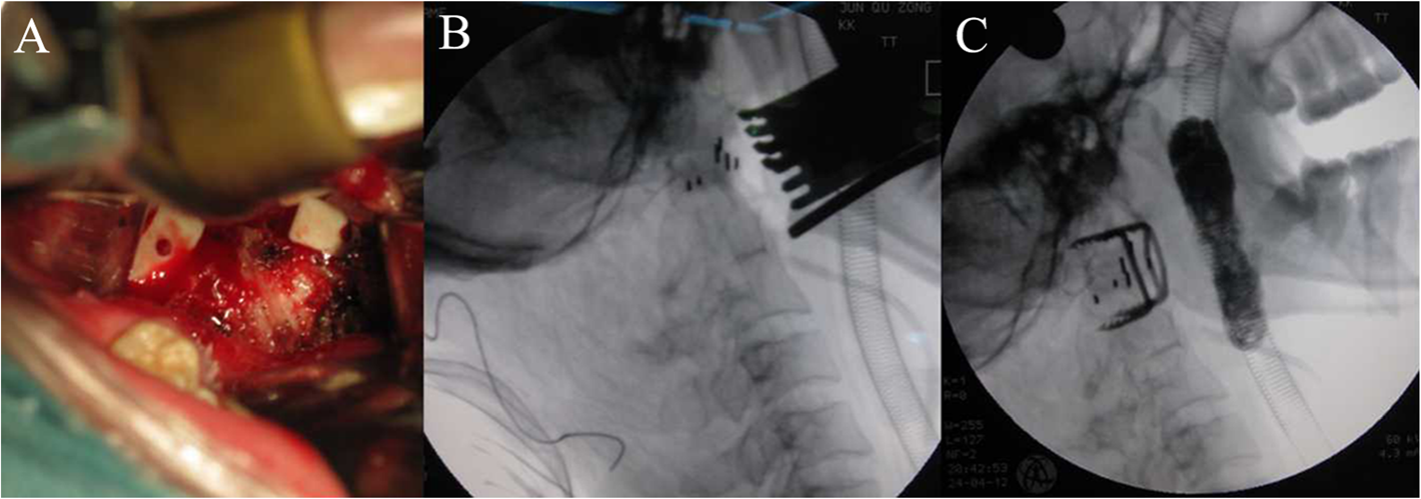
Intraoperative procedures. a Photograph after the placement of bilateral intraarticular cages. b Intraoperative X-ray after the implantation of bilateral intraarticular cages showed satisfactory atlantoaxial reduction. C. Intraoperative X-ray after the implantation of C-JAWS showed well position of devices
